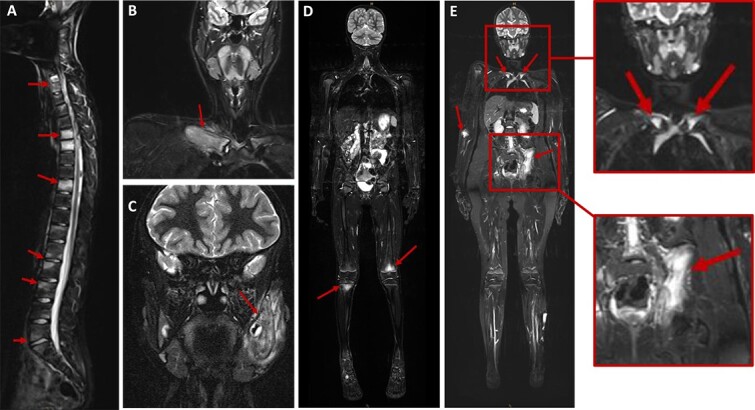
MRI findings in patients with CNO. Whole body (WB) MRI can detect inflammation before structural changes are present. (A) Sagittal TIRM sequences showing total and focal hyperintensities in multiple vertebral bodies. (B) Coronal TIRM sequences with hyperintense thickening of the left mandible and surrounding inflammatory soft tissue. (C) Coronal TIRM sequences revealing hyperintense thickening of the right clavicle and surrounding inflammatory soft tissue. (D) TIRM-sequences showing typical hyperintense lesions in the distal femoral metaphysis (right) and proximal tibial metaphysis (left). (E) TIRM-sequences revealing CNO-typical hyperintense lesions in the distal humeral metaphysis (right), in the medial claviculae and os ilium (left). Boxes on the right show enlarged sections displaying inflammatory activity affecting with claviculae (top) and os ilium (bottom).
